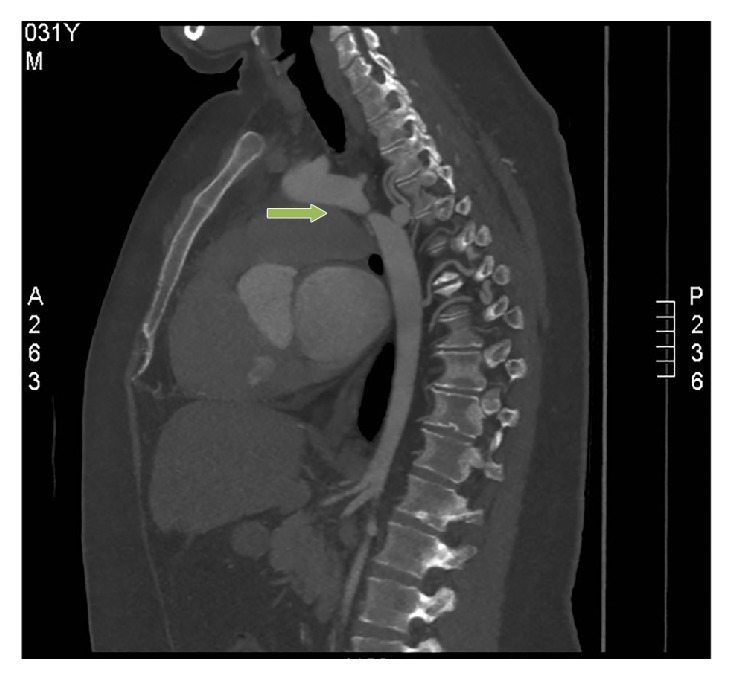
CT of left upper limb showing coarctation of the aorta at the isthmus.
